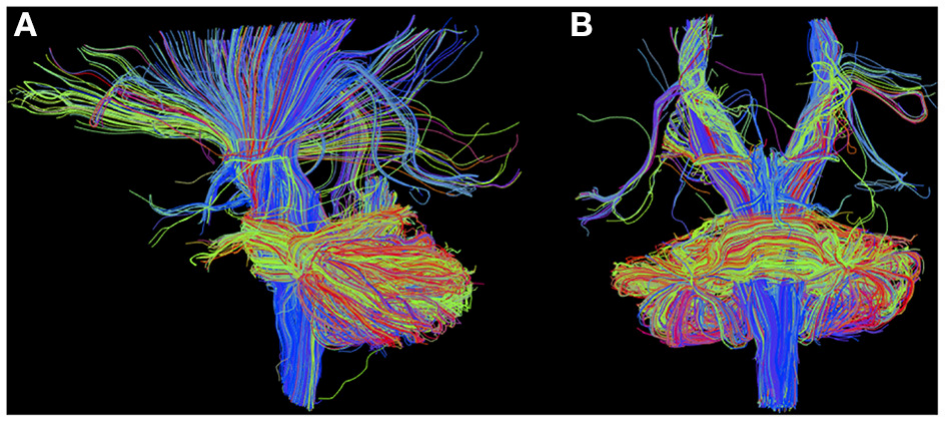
Diffusion MRI with region-of-interest-based deterministic tractography using TrackVis in sagittal (A) and coronal (B) view, rendered as described with MR data from the protocol pilot run (3T MAGNETOM Prisma, Brain Imaging Center Frankfurt) obtained from a healthy 32-year-old male test subject.
